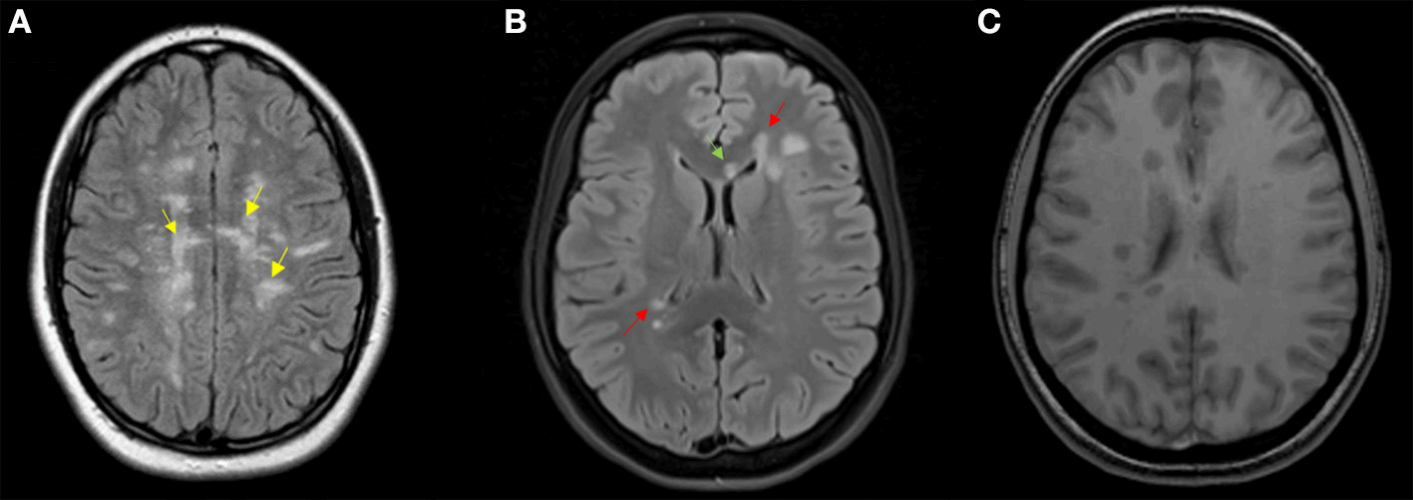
Representative MRI scans. (A) Axial T2 Flair MRI of a 15-year-old girl featuring Dawson's finger lesions (yellow arrows); (B) axial T2 Flair MRI, and (C) axial T1 MRI of a 16-year-old girl showing periventricular (red arrows) and corpus callosum (green arrow) lesions, and T1 hypointense lesions.
